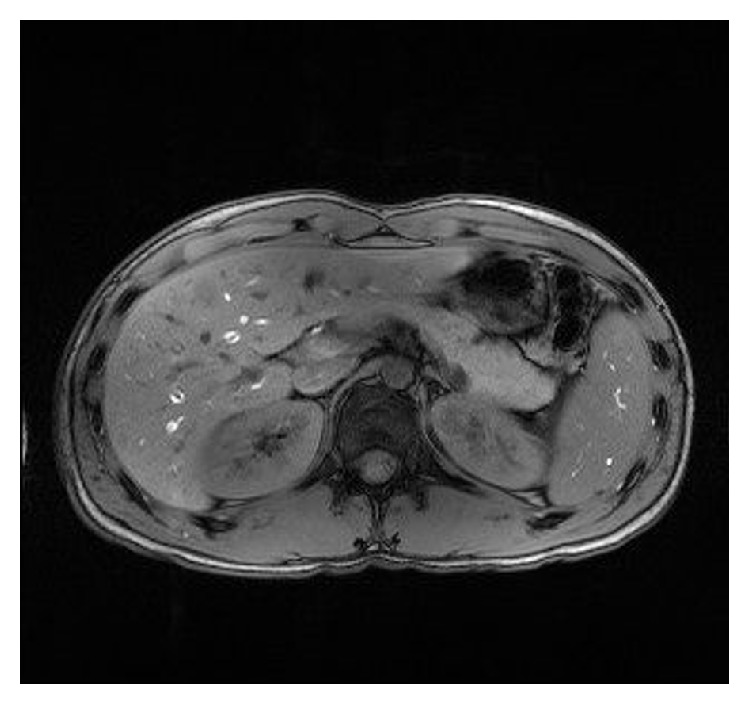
Follow-up MRI with most likely fluid-filled hepatic and splenic lesions.
